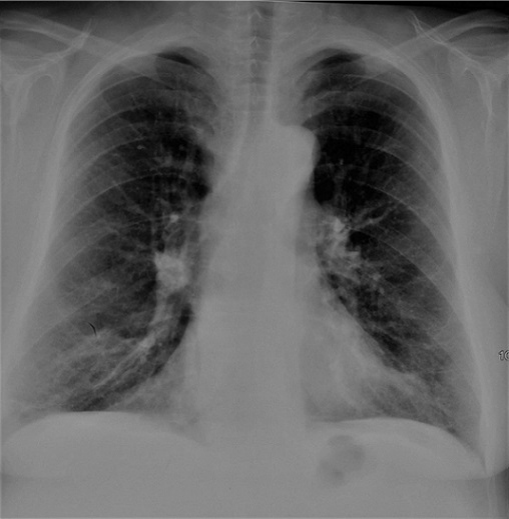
Radiographie thoracique de face montrant des signes de distension thoracique et un épaississement bronchique
